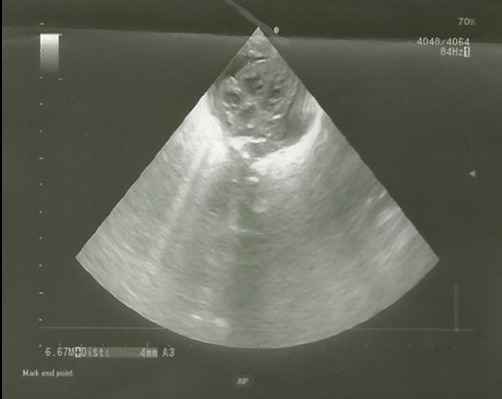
Reduction of pericardial effusion on echocardiogram immediately after pericardiocentesis
